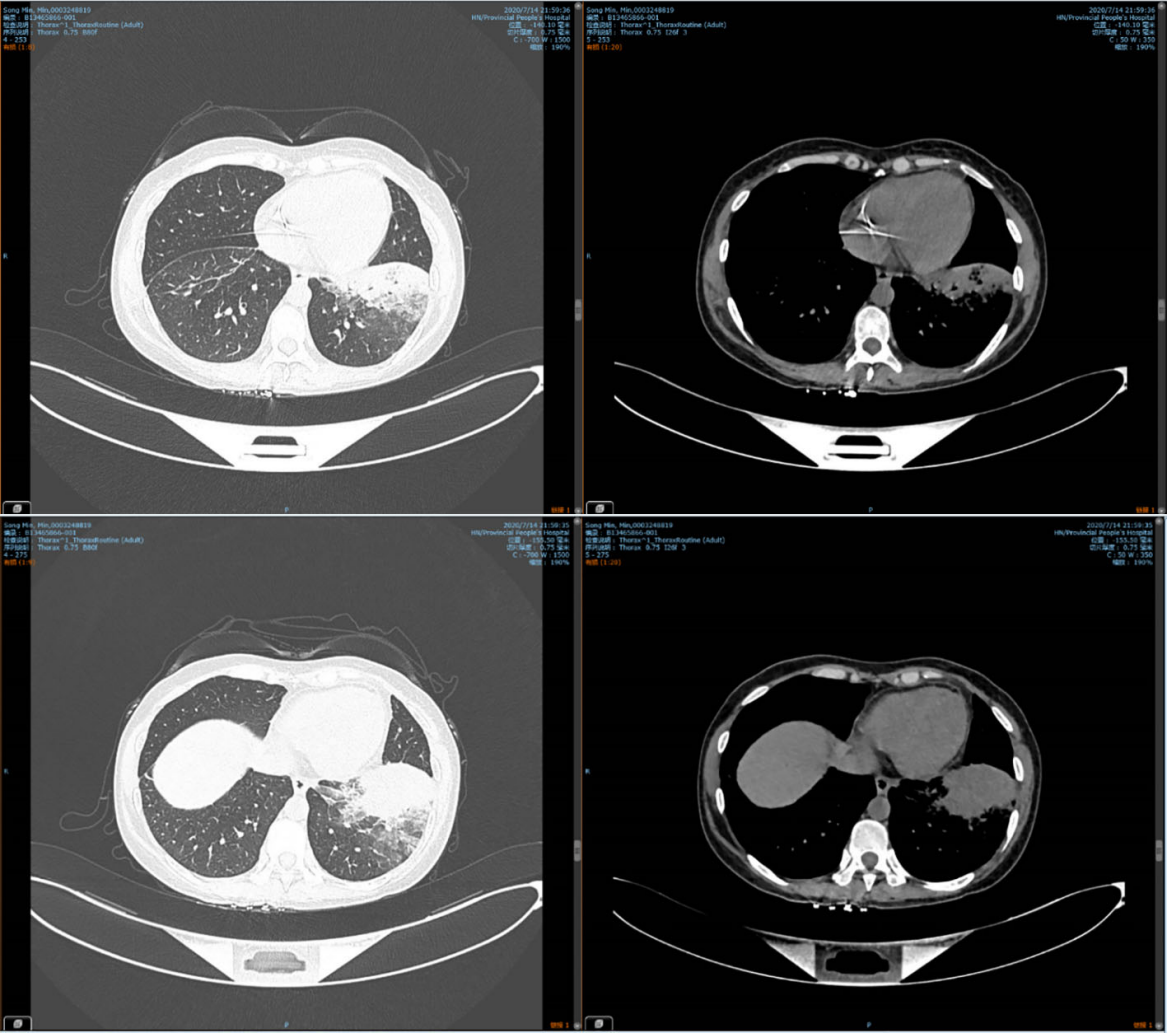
Chest CT on July 14, 2020, showing consolidation in the left lower lung.
